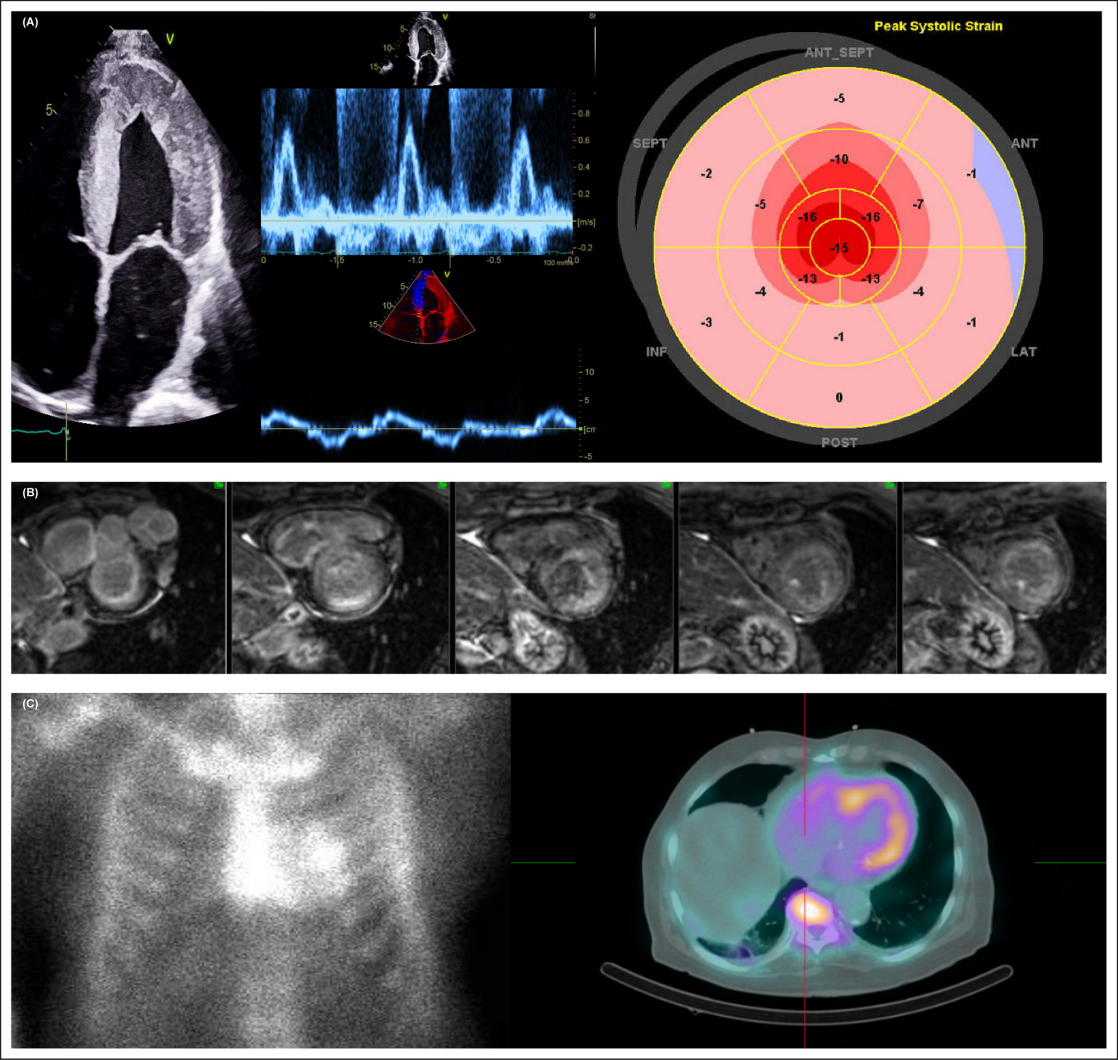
Multimodality imaging approach for cardiac amyloidosis (CA). (A) Increased left ventricular (LV) wall and interatrial thickness with sparkling texture are typically found in patients with CA (left). Evidence of restrictive LV filling, rapid E-wave deceleration time, high E/A ratio on mitral inflow Doppler, and low myocardial relaxation velocities on tissue Doppler imaging (middle). Corresponding “bullseye” map of the longitudinal strain pattern of the LV with a “cherry on top” sign (right). (B) Cardiac magnetic resonance imaging shows characteristic imaging of CA with diffuse and subendocardial late gadolinium enhancement. (C)
